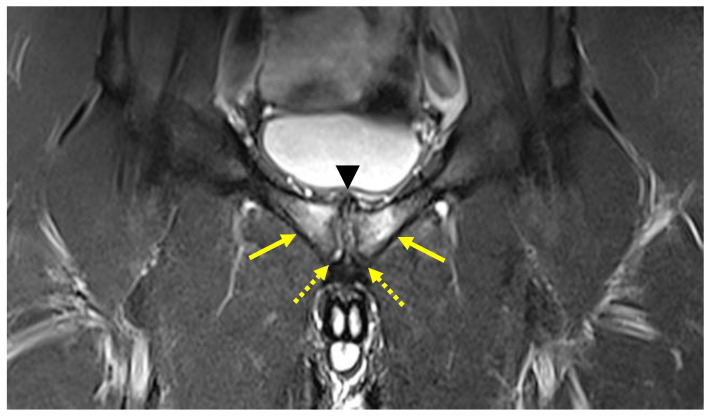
MRI in coronal STIR view showing central symphyseal disc protrusion (arrowhead), bilateral bone marrow oedema (arrows) and irregularities of the symphysis (dotted arrows). These radiological signs indicate severe pubic osteopathy.
